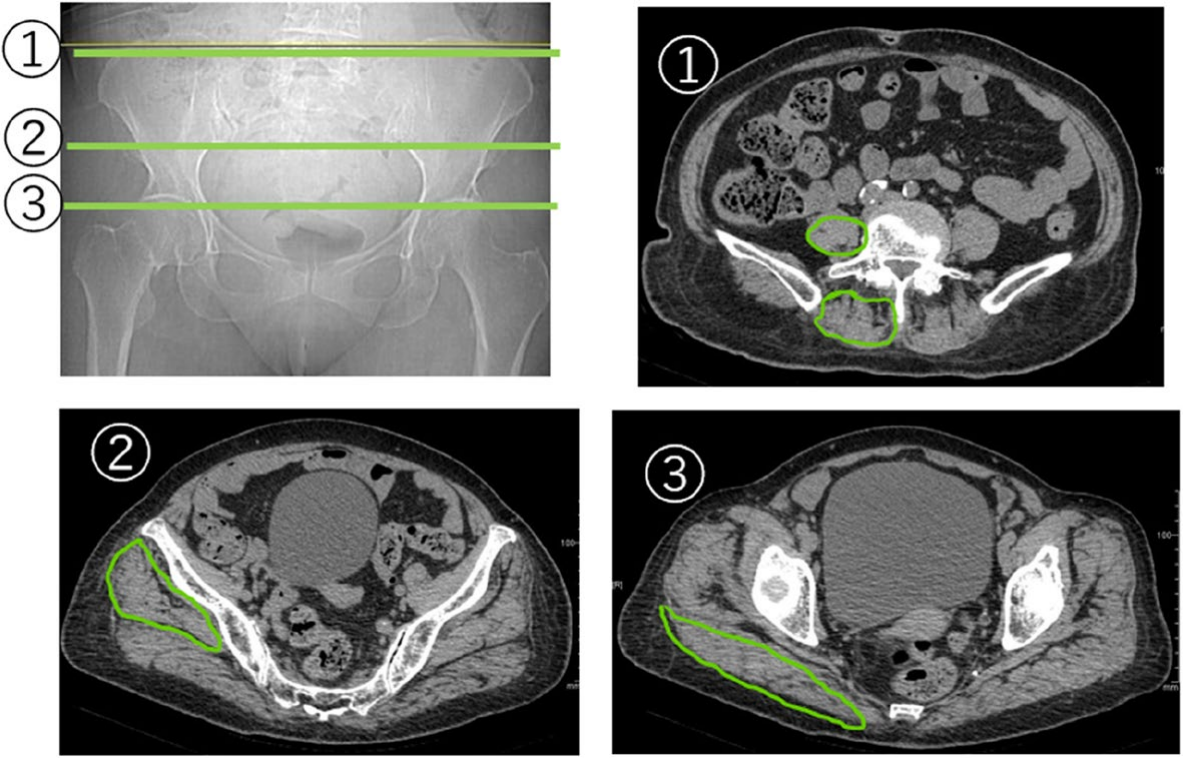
Axial CT scan slices and the measurement of muscle cross-sectional area. (1) Superior end of the L5 vertebra – paraspinous /psoas muscles; (2) inferior end of the sacroiliac joint – gluteus maximus muscle; and (3) superior end of the femoral head – gluteus medius muscle
